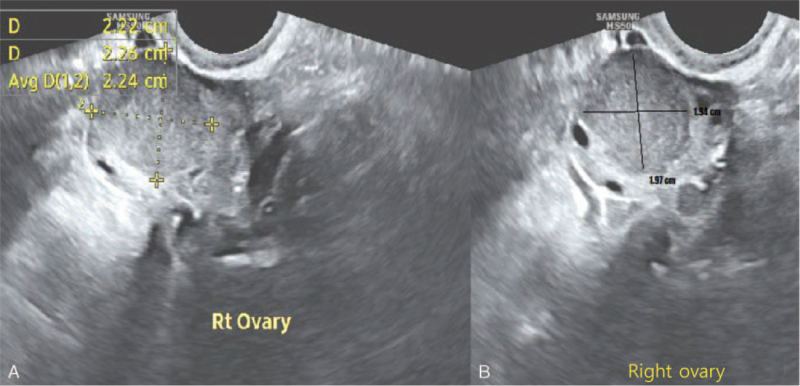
Ultrasonographic findings taken at local clinic 2 yrs ago. (A) A 20 × 37-mm sized right ovarian mass was incidentally on an ultrasound taken at local clinic 2 yrs ago. (B) The mass was thought to be ovarian endometrioma.
